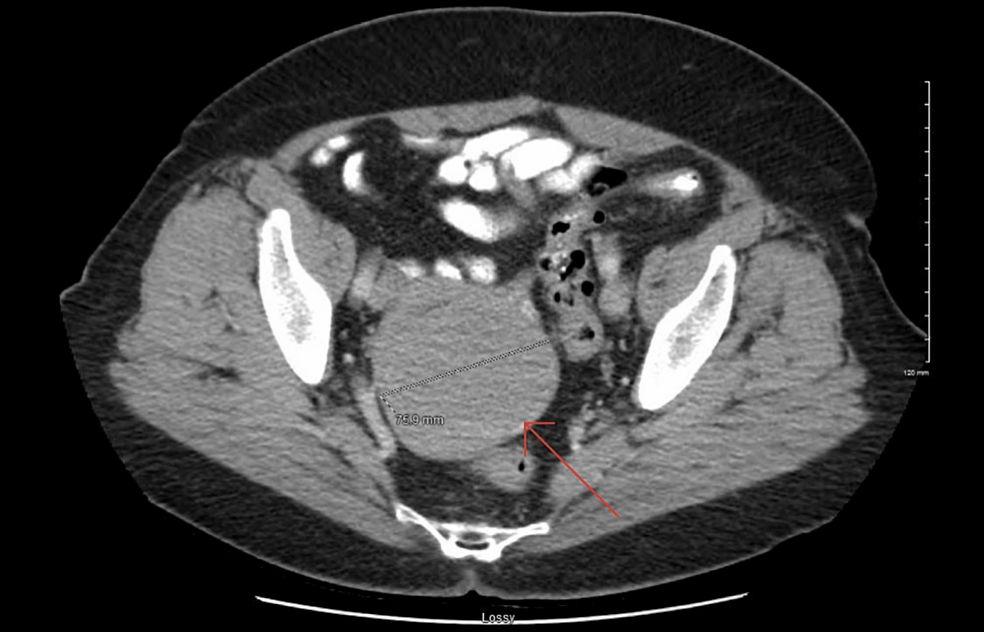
Axial CT scan of the abdomen/pelvis shows a large uterine fibroid (red arrow)
